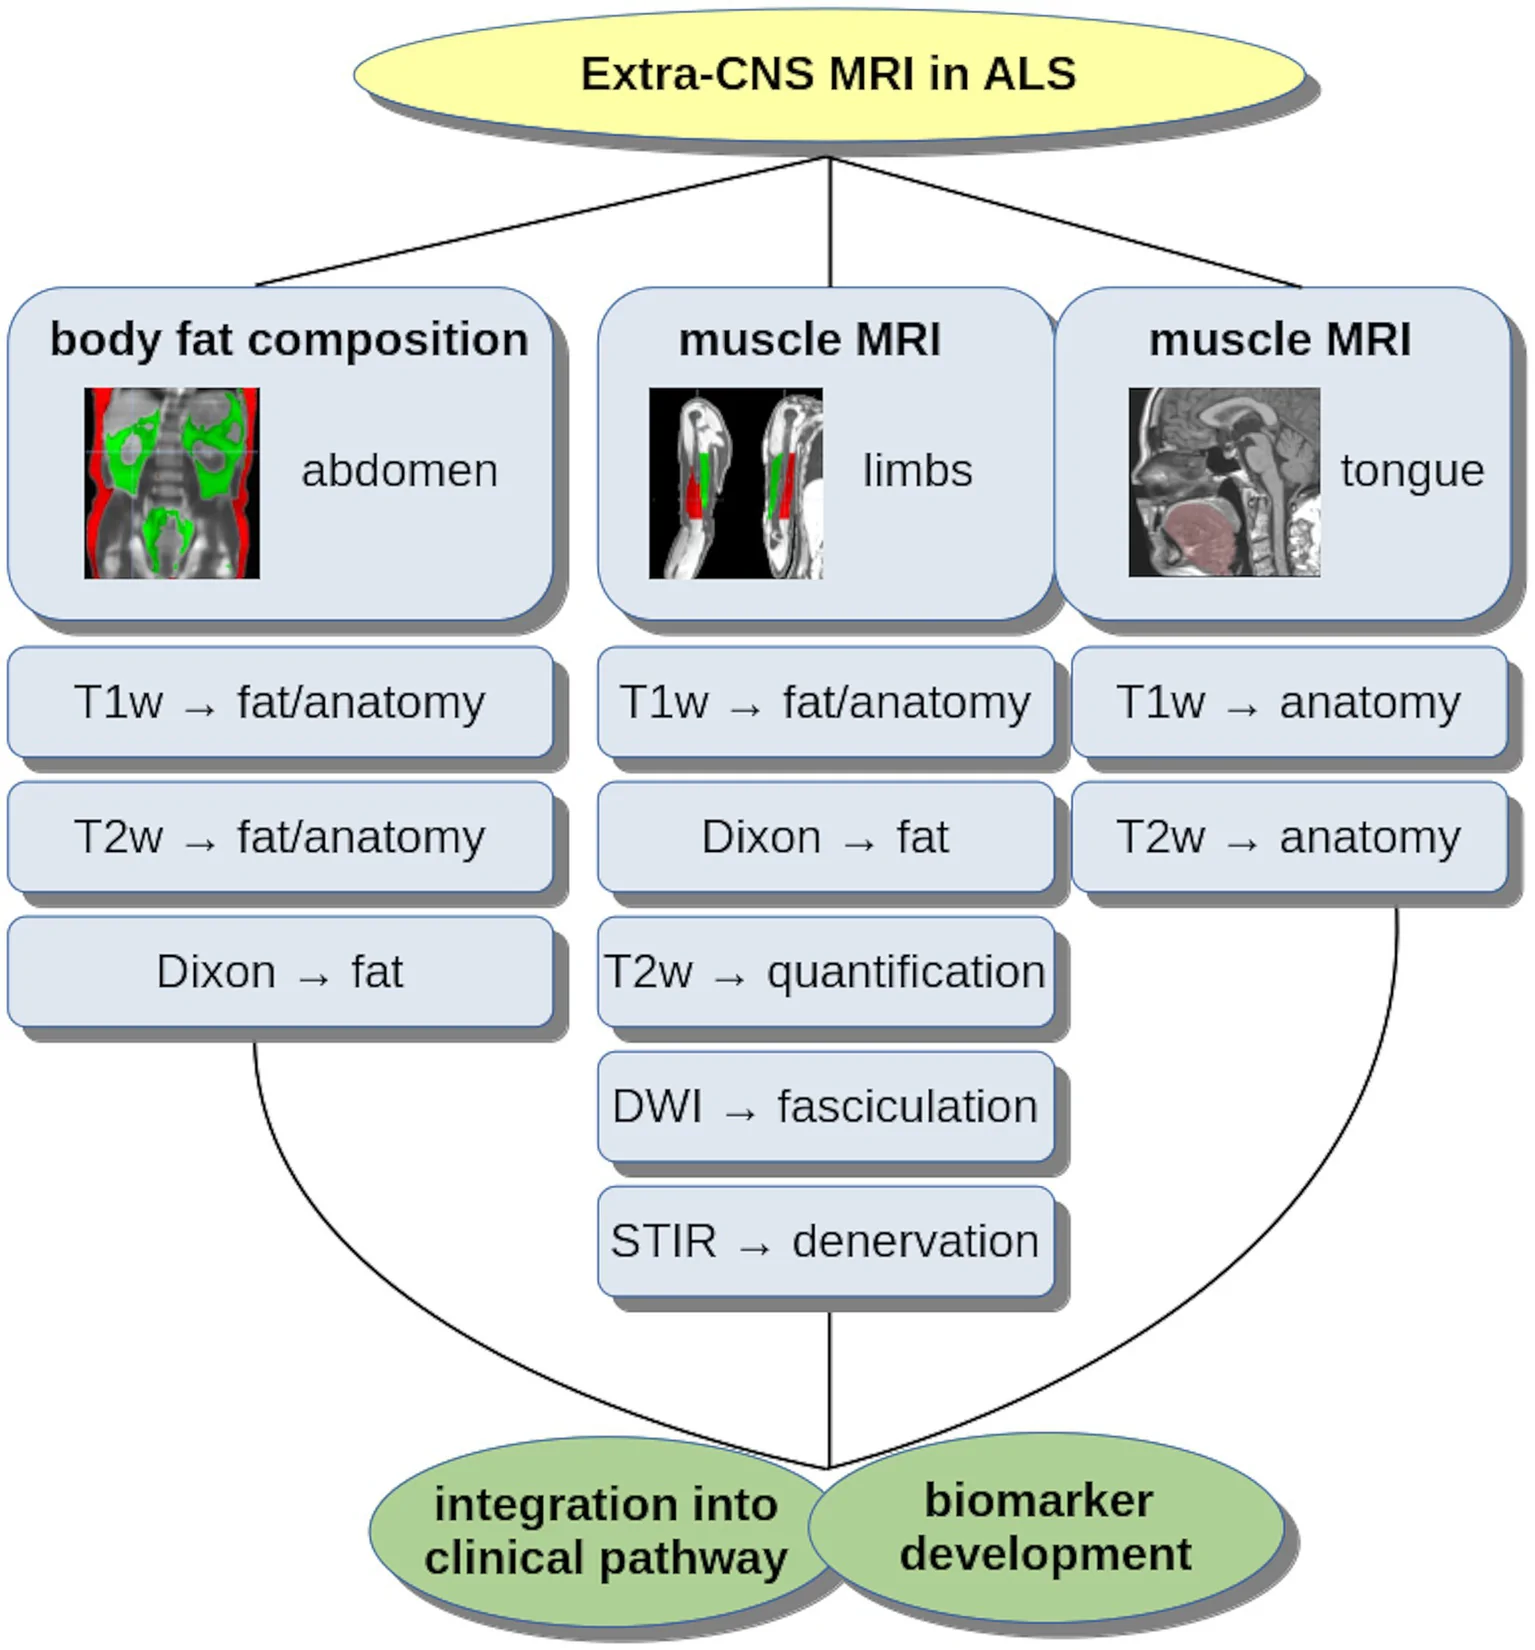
The role of extra-CNS imaging in ALS. MRI of body fat compartments and muscle MRI including limb and tongue MRI, respectively, are promising techniques to be integrated into clinical pathway and for biomarker development. CNS, central nervous system; MRI, magnetic resonance imaging; ALS, amyotrophic lateral sclerosis; DWI, diffusion weighted imaging; STIR, Short Tau Inversion Recovery.
